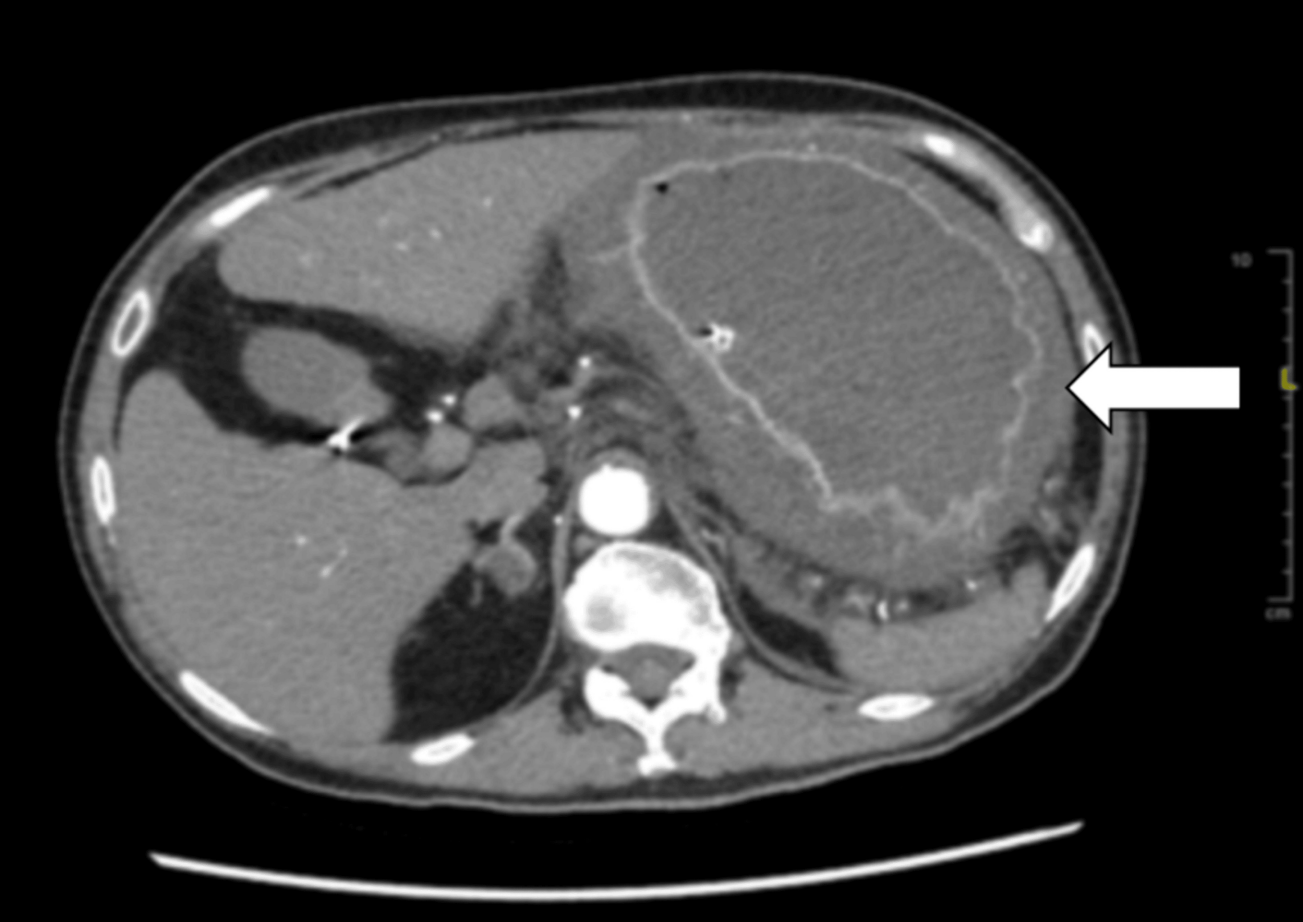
Diffuse thickening of the gastric wall with surrounding fat stranding detailing in the abdomen contrast-enhanced CT scan axial view (white arrow).
